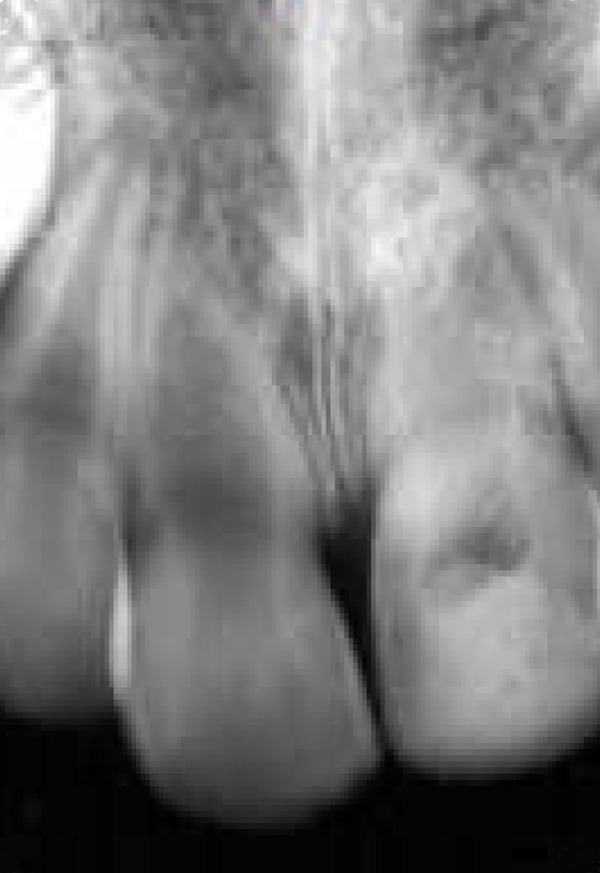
Radiograph taken one year after avulsion
